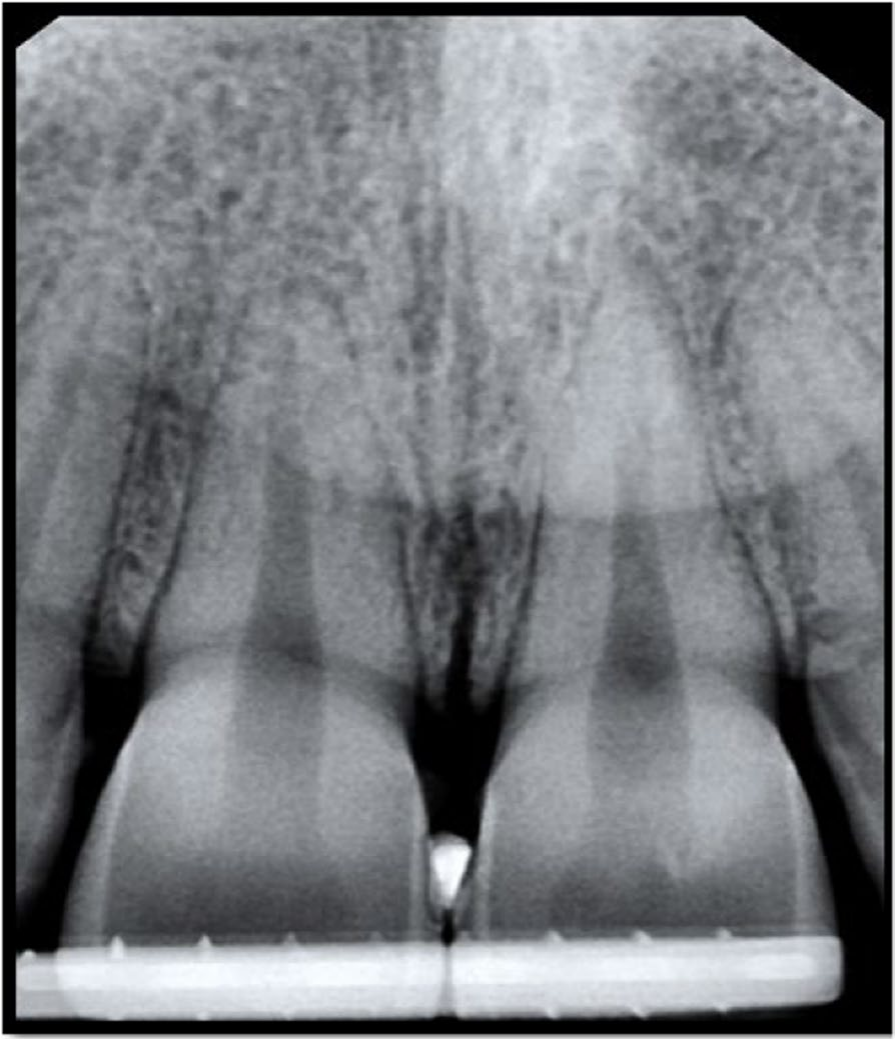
Digital periapical radiographs with a reproducible alignment device for standardization while radiopaque material placed at papilla tip
